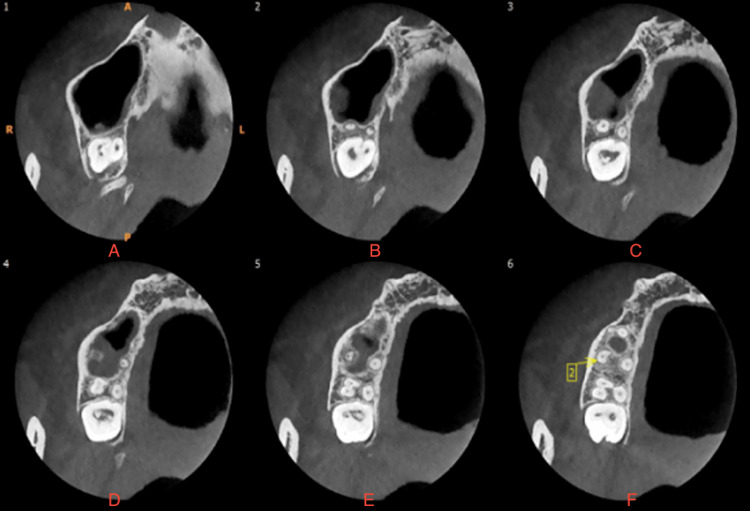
CBCT systems transverse section of teeth: (A-F) CBCT images of different levels from the apical direction; 2: a single apical opening of the root canal after fusion of the mesiobuccal and distobuccal roots. CBCT, cone-beam computed tomography
